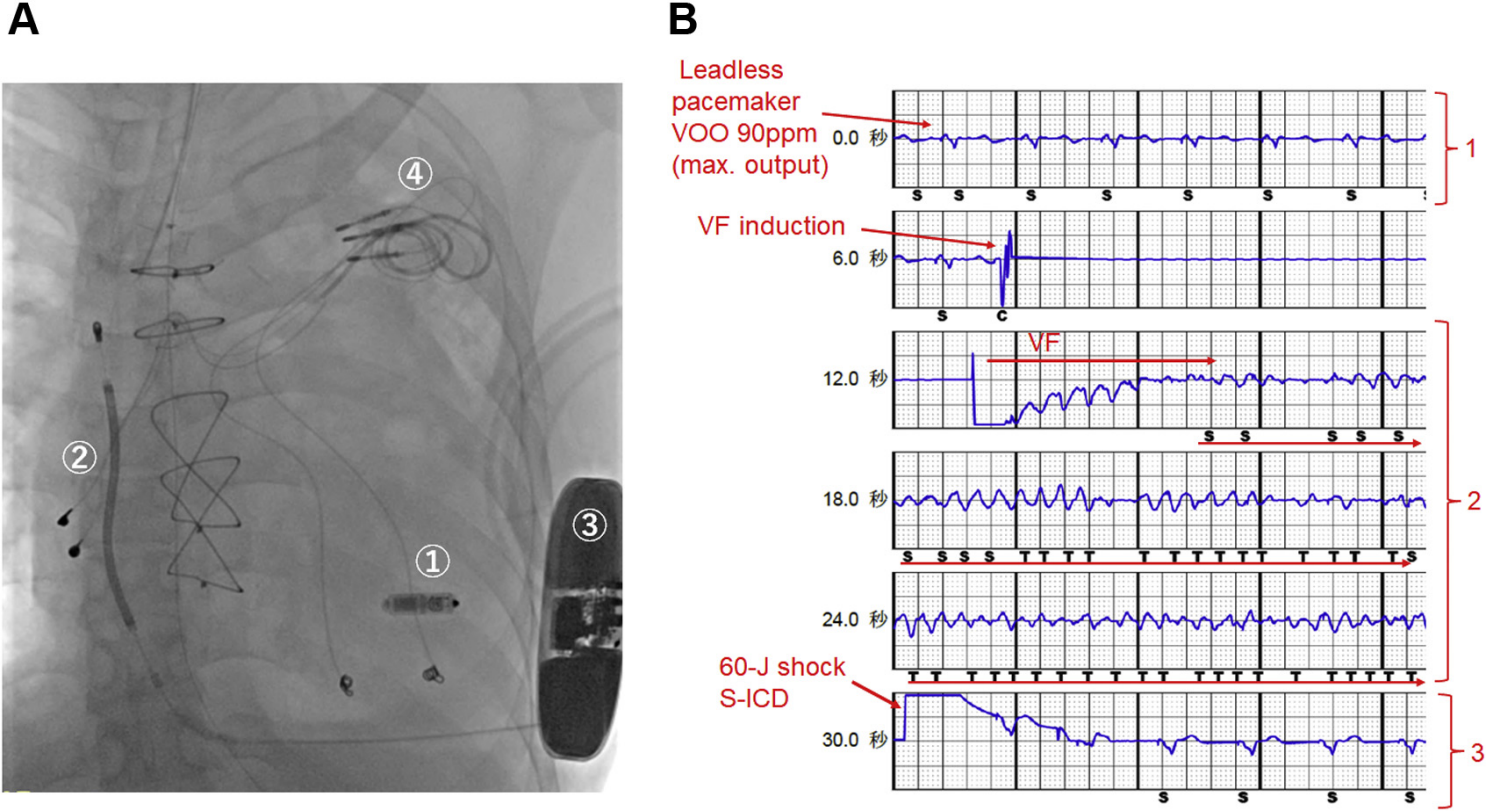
Implantation of S-ICD (A) Implantation of the subcutaneous implantable cardioverter-defibrillator (S-ICD): *1,* leadless pacemaker; *2,* the right parasternal position of the subcutaneous implantable cardioverter-defibrillator lead, *3,* subcutaneous implantable cardioverter-defibrillator generator; *4,* removal of the existing pacemaker. (B) Ventricular fibrillation (VF) induction test: *1,* ventricular fibrillation was induced during the paced rhythm (VOO) at maximal output from the leadless pacemaker; *2,* neither oversensing of the pacing spike nor undersensing of the ventricular fibrillation was observed; *3,* a 60-J shock from the subcutaneous implantable cardioverter-defibrillator terminated the ventricular fibrillation.
